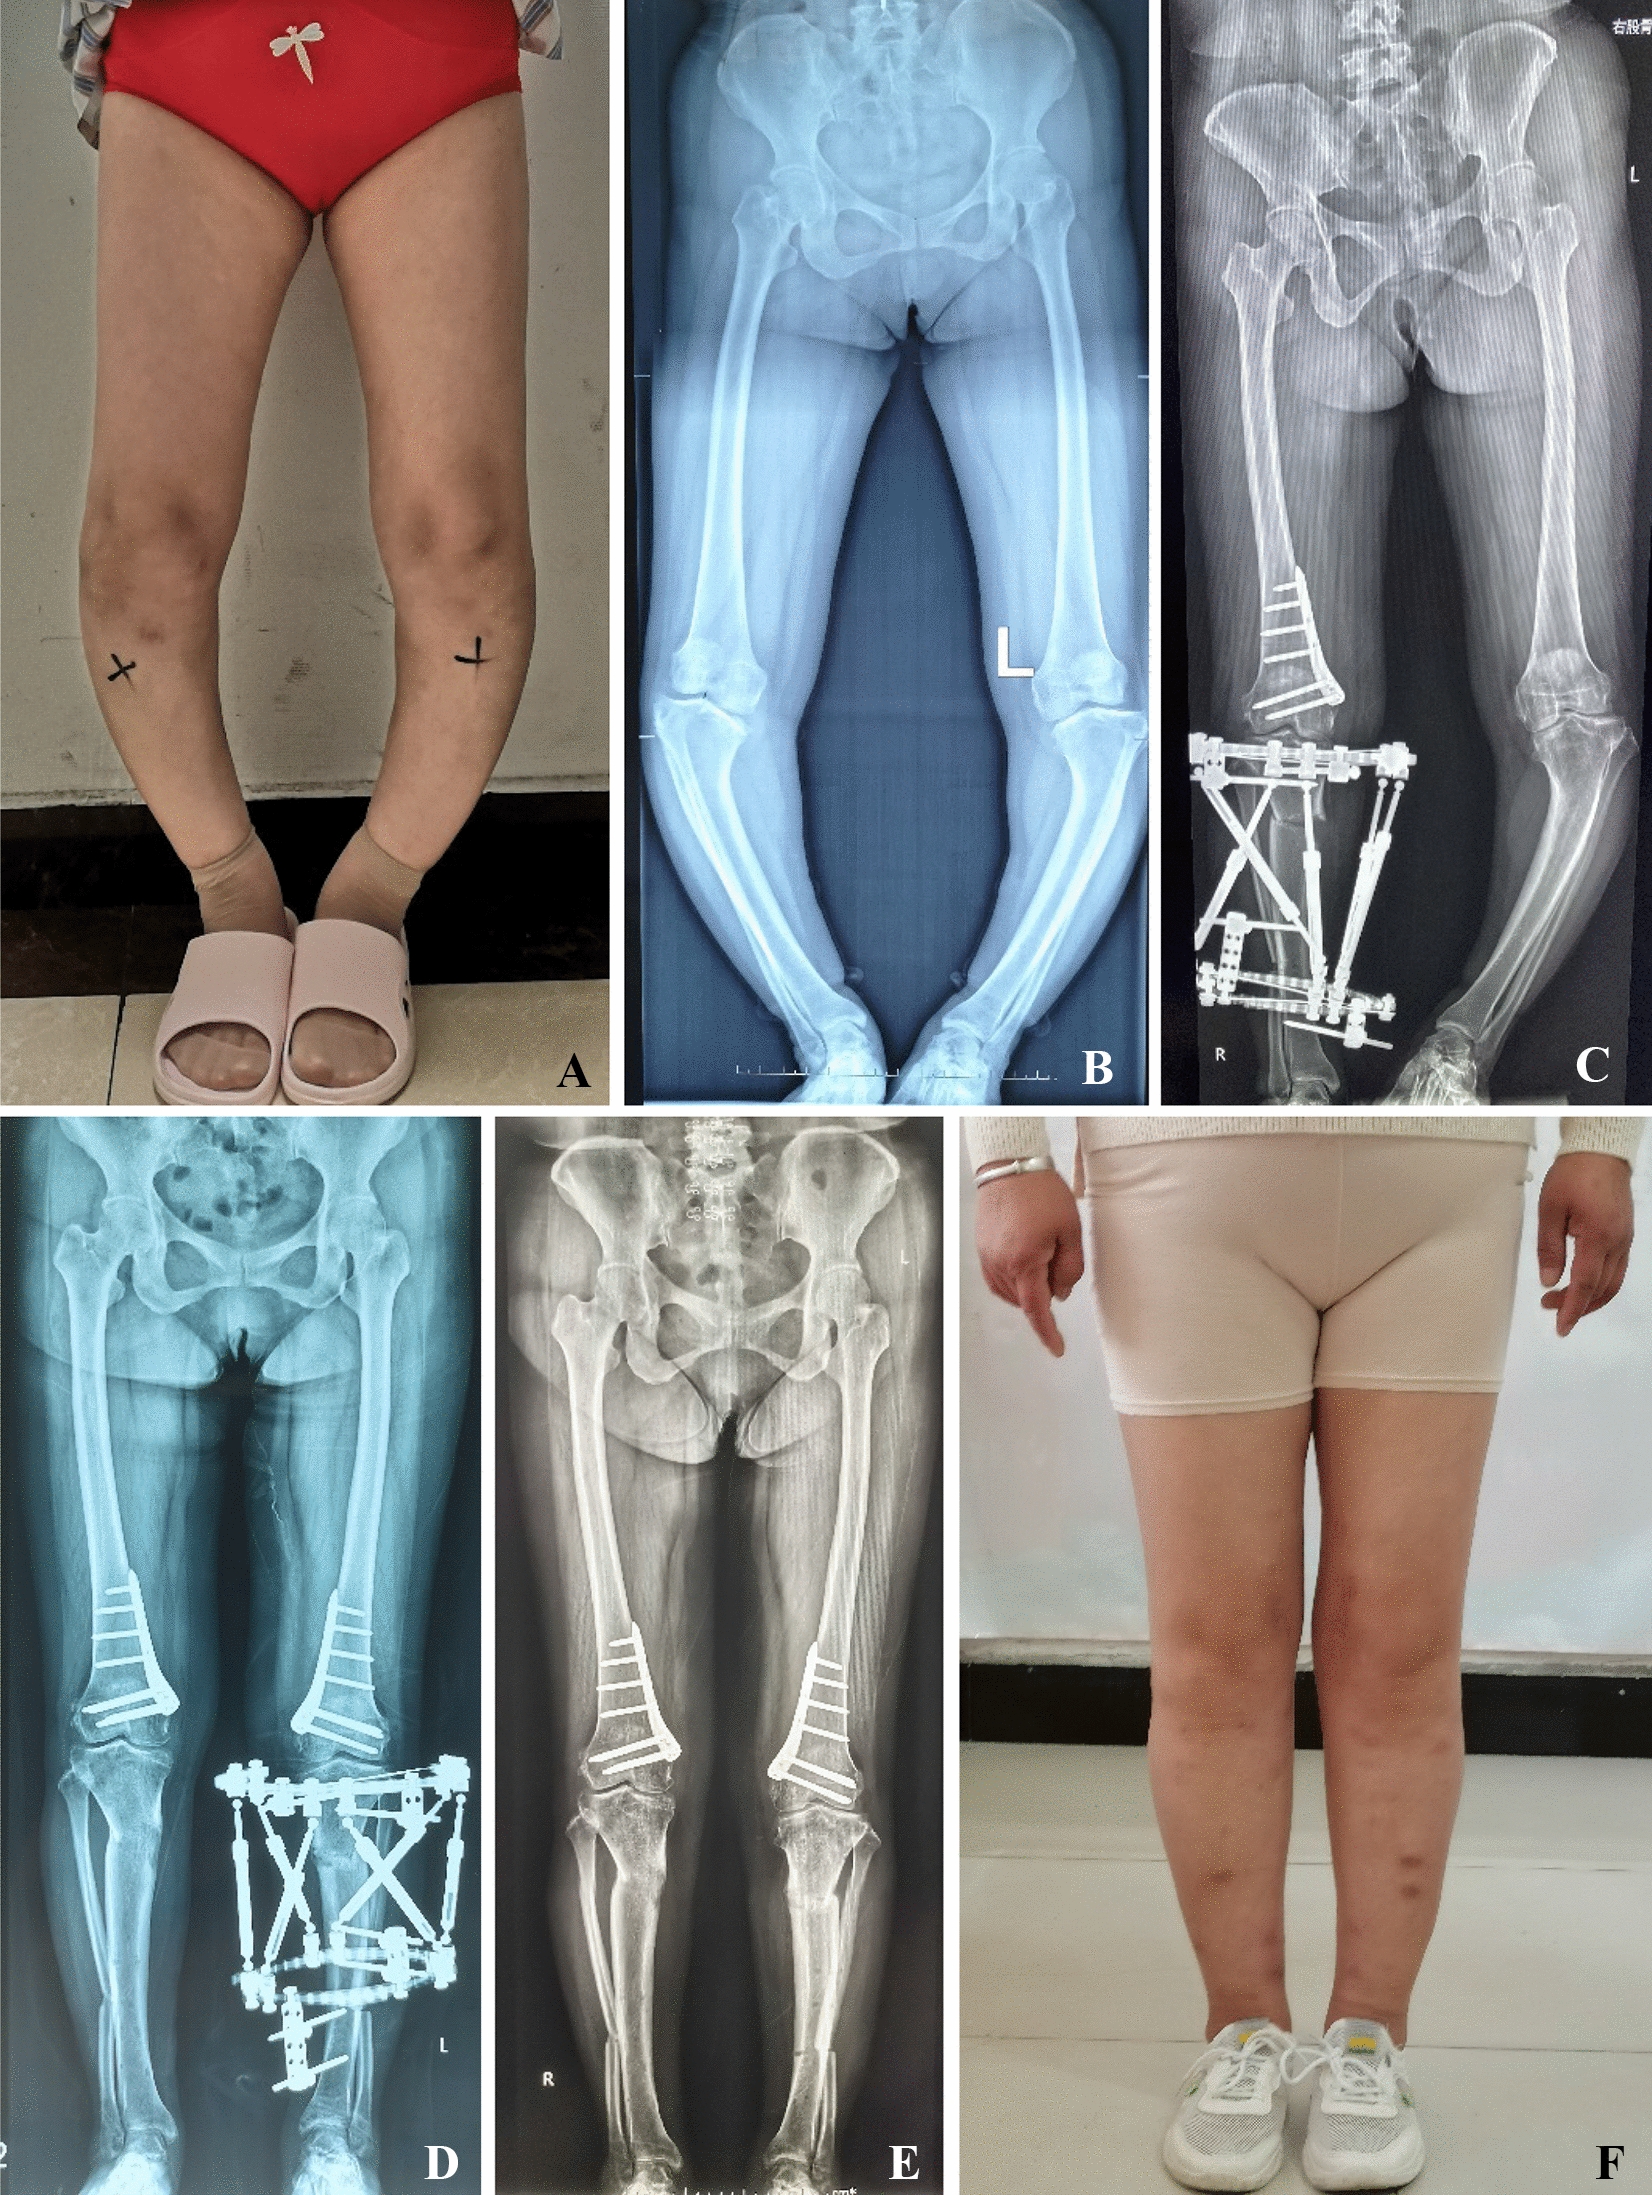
A 38-year-old female with juvenile Blount disease and untreated residual genu varus deformity. A, B Preoperative appearance and radiograph of lower limbs. C One-stage right tibial osteotomy QSF fixation and distal femoral osteotomy with internal fixation. The external frame was adjusted for 30 days, and the osteotomy site healed 15 weeks after the adjustment. At this point, the external fixator was removed. D Surgery was conducted on the left lower limb after 6 months. E, F Appearance and radiograph of the patient's lower extremities after the frames were removed
